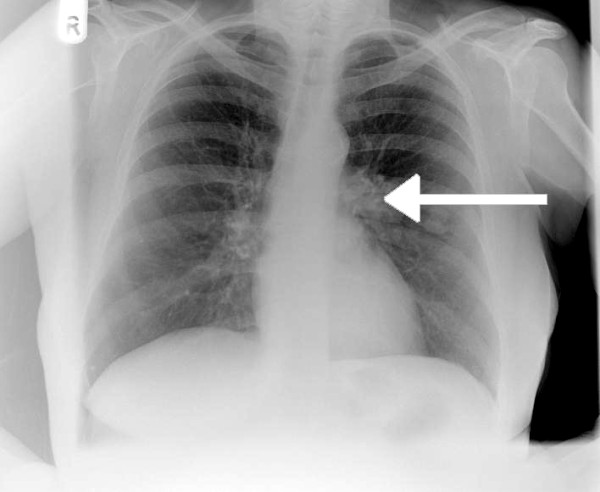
Chest radiograph showing left hilar abnormality (arrow) and opacity in the left mid zone.
